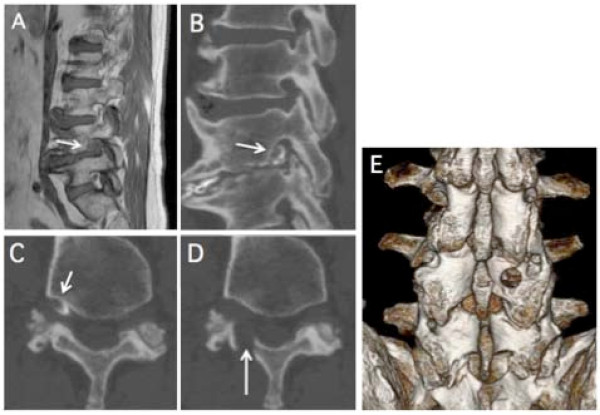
Case 6. Images from a 56-year-old male who presented with severe right leg pain. A: Para-sagittal MRI imaging (T1) showed the obliteration of the normal increased signal intensity fat (arrow). B,C: Para-sagittal and axial reconstruction CT-discograms showed an intraforaminal herniated disc (arrow) at the L5-S1 level. D: A CT-discogram obtained at L5-S1 showing partial resection in the L4 lamina (arrow). E: Postoperative 3-D reconstruction showing the oval fenestration in the L4 lamina.
